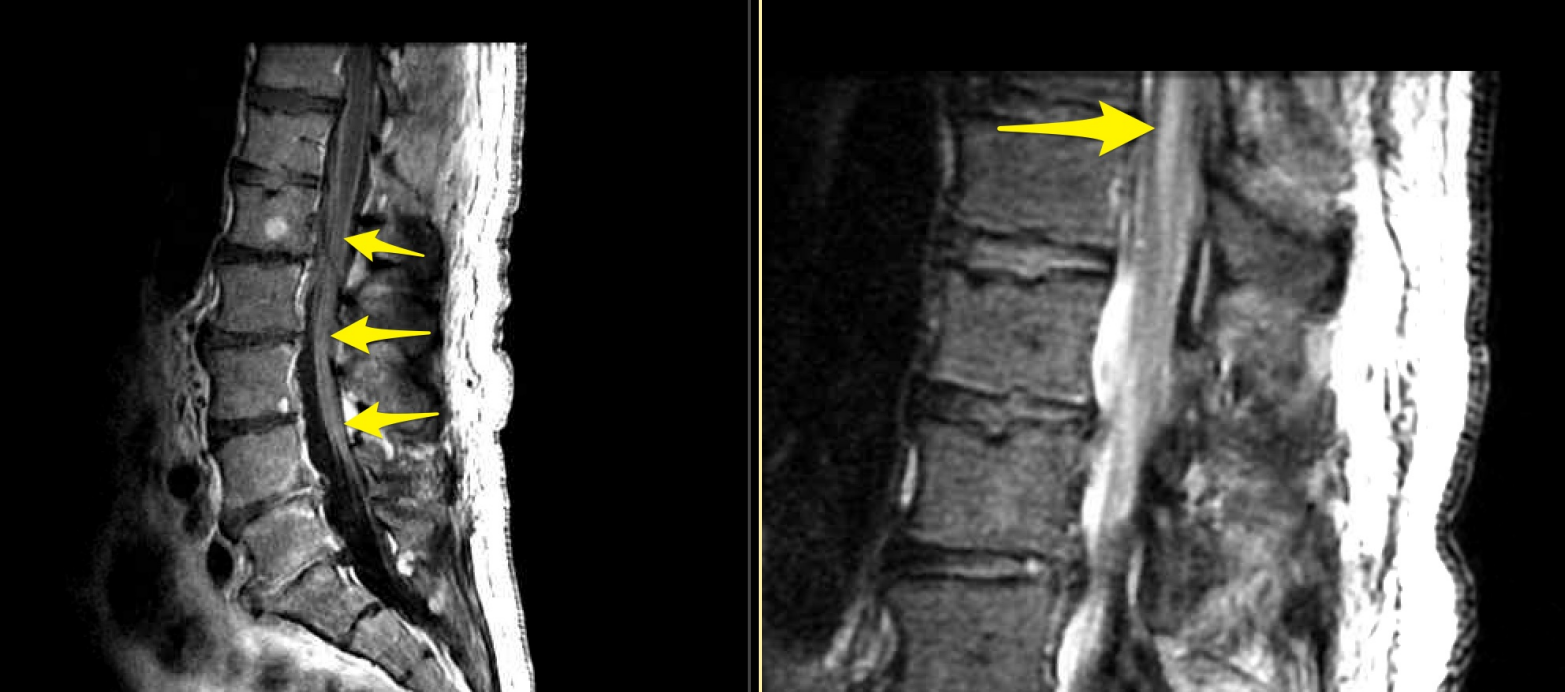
T1 post-contrast sagittal lumbar MRI showing cauda equina enhancement and T2 sagittal lumbar MRI showing conus medullaris edema. MRI: magnetic resonance imaging
